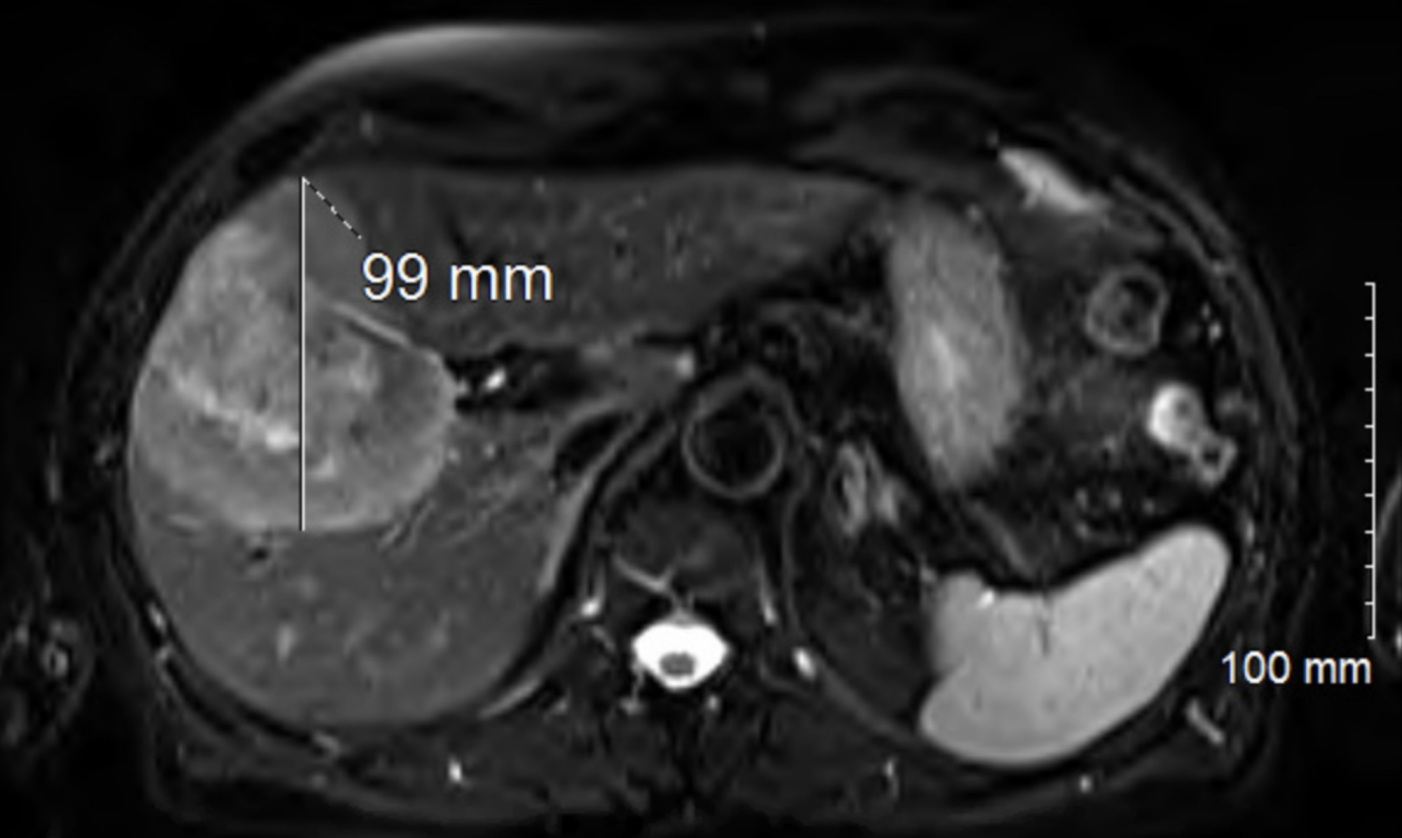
MRI abdomen showing enhancing mass with necrotic center
